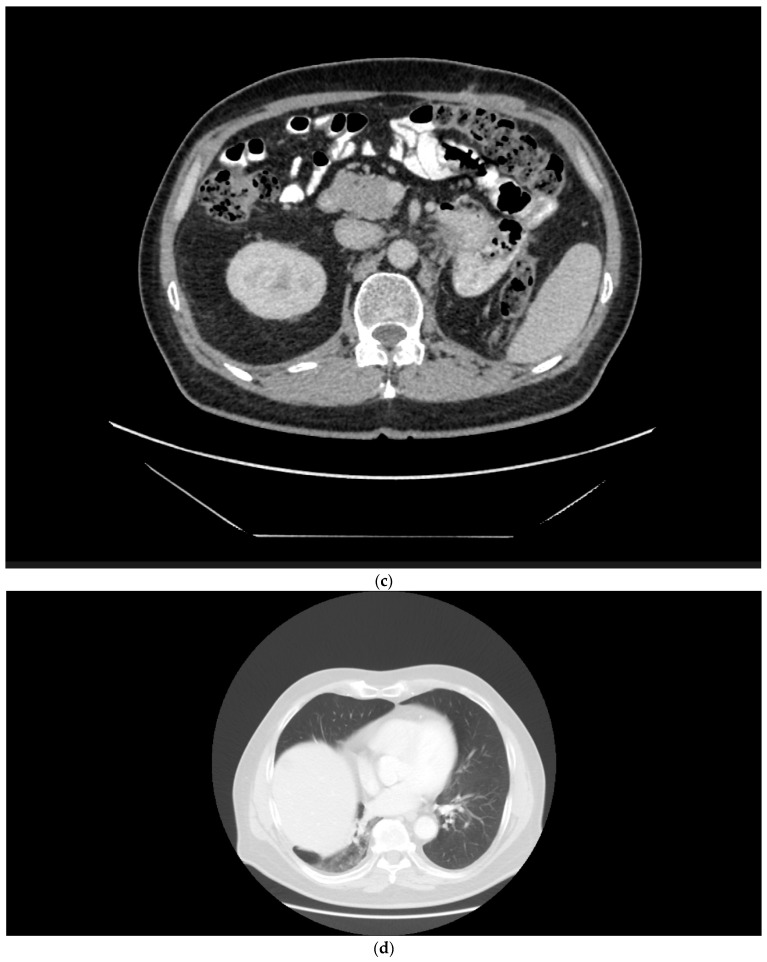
(a) Patient 2, pretreatment CT of the renal tumor. (b) Patient 2, pretreatment CT of the chest demonstrating lung metastases. (c) Patient 2, a large mass in the retroperitoneum. (d) Patient 2, major partial response after the combination regimen of ipilimumab with nivolumab.
